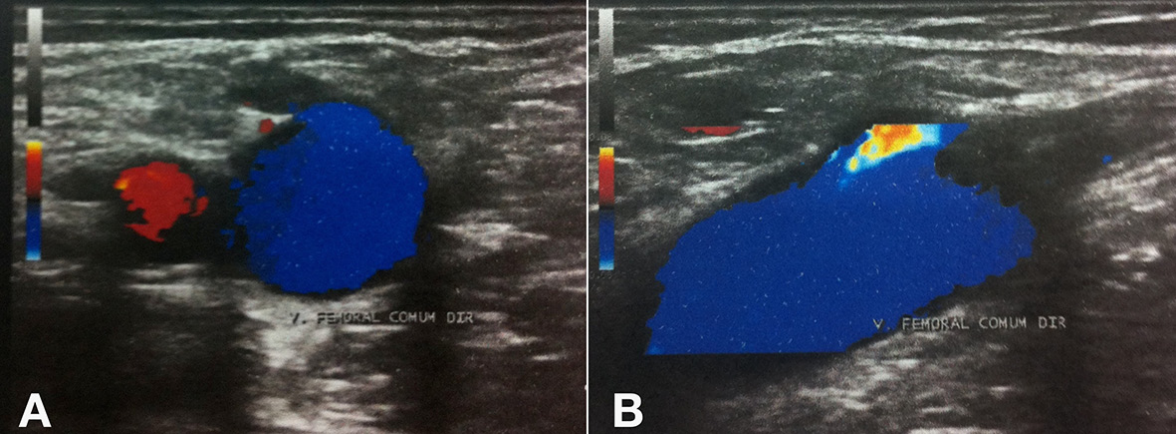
Eco-Doppler de controle após o sétimo dia de tratamento com rivaroxabana. (A) Imagem em modo color com transdutor na transversal; (B) Imagem em modo color com transdutor na longitudinal.
